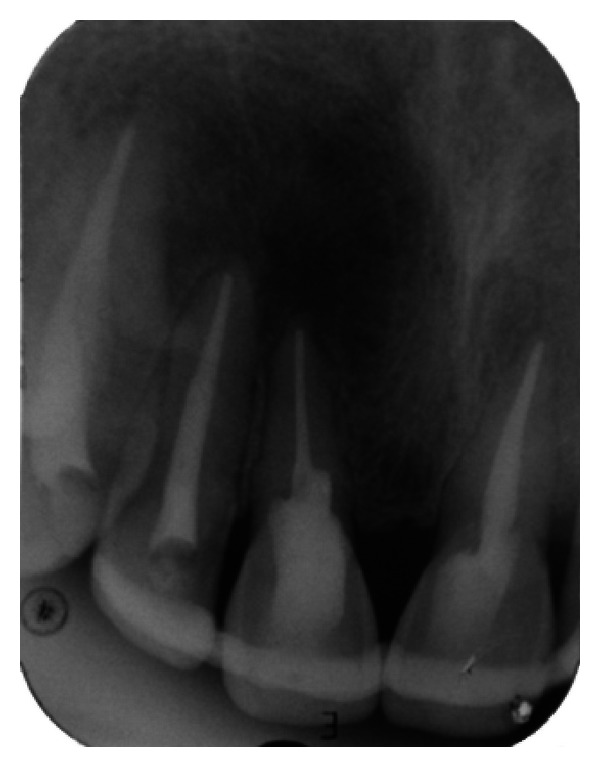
6-month followup IOPA radiograph.
